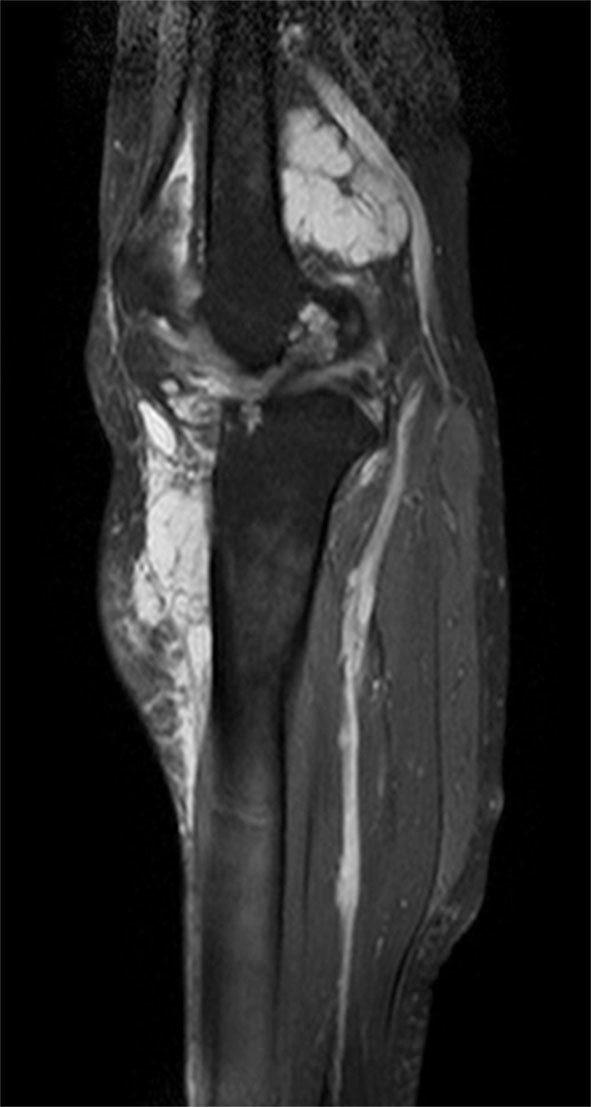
Sagittal short tau inversion-recovery MRI of the knee showing involvement of Hoffa’s fat pad and the femoral intraosseous component.
